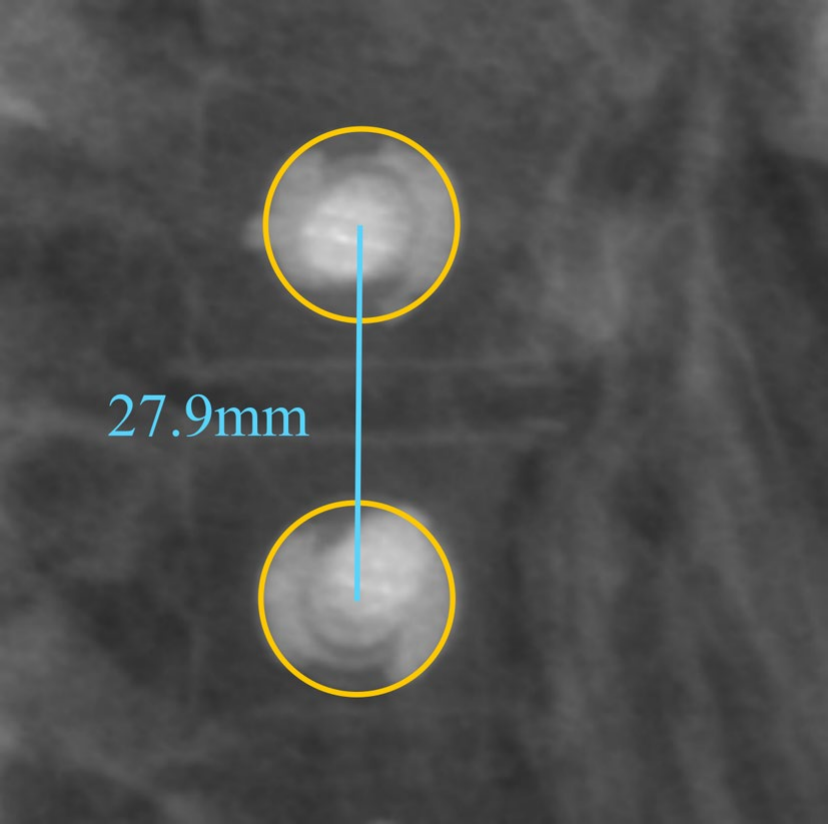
Measurement of inter-screw distance on sagittal radiographs. Centers of the orange circles surrounding the two adjacent screw heads were used to draw the inter-screw distance line
